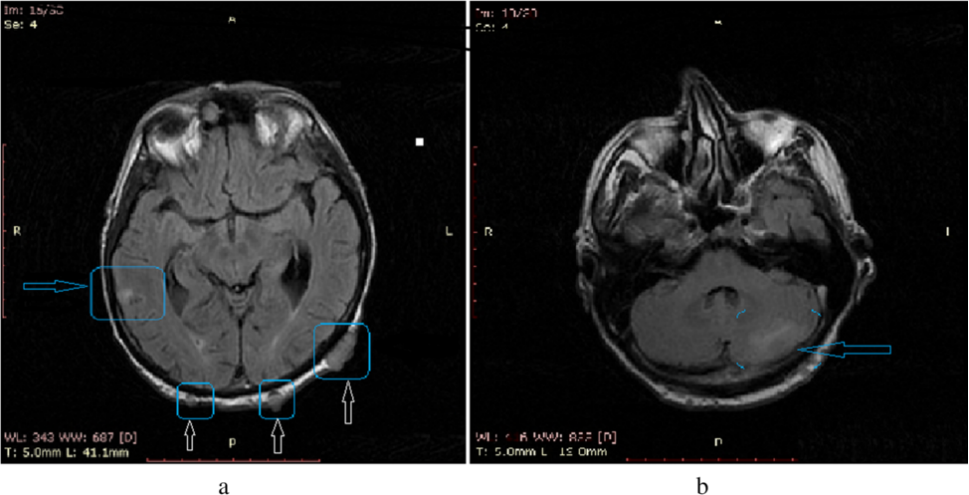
(a, b) Magnetic resonance imaging of metastases located in the cortex of the temporal lobe, scalp (a) and in the left cerebellar hemisphere (b).
